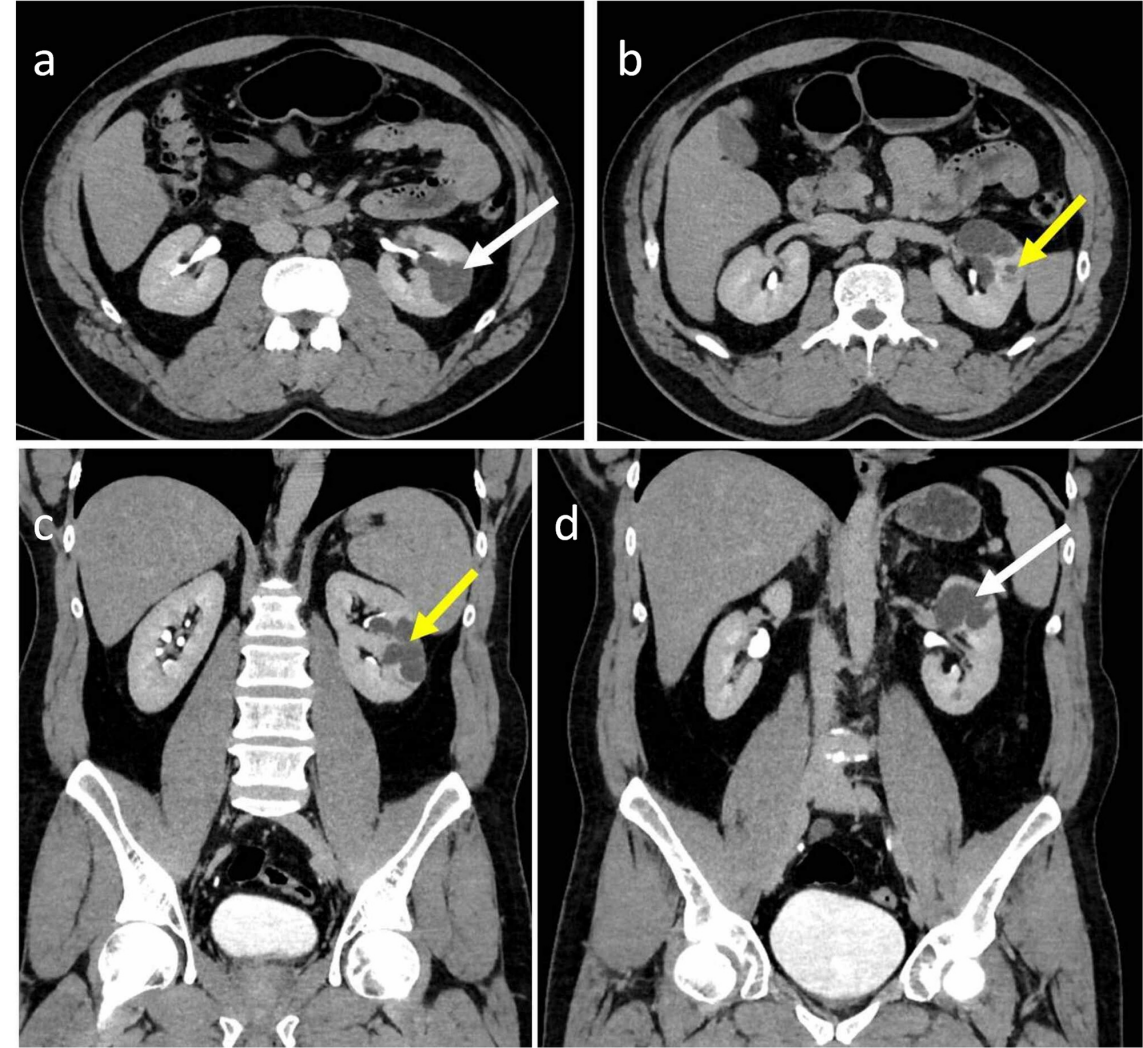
30-year-old male with left parapelvic and intrarenal lymphangiectasia Contrast-enhanced CT of the abdomen in the venous phase in axial (a, b) and coronal (c, d) planes demonstrates left parapelvic (white arrows) and intrarenal lymphangiectasia (yellow arrows) CT: computed tomography
